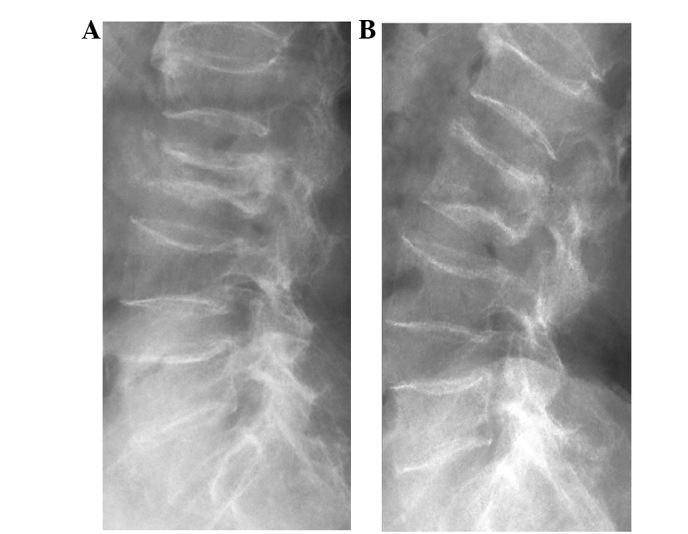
X-ray views of an 80-year-old female patient suffering from Kümmell’s disease at L3. (A) Standing flexion lateral X-ray view showed L3 vertebra compression. (B) Standing extension lateral X-ray view showed L3 vertebra height increased.
